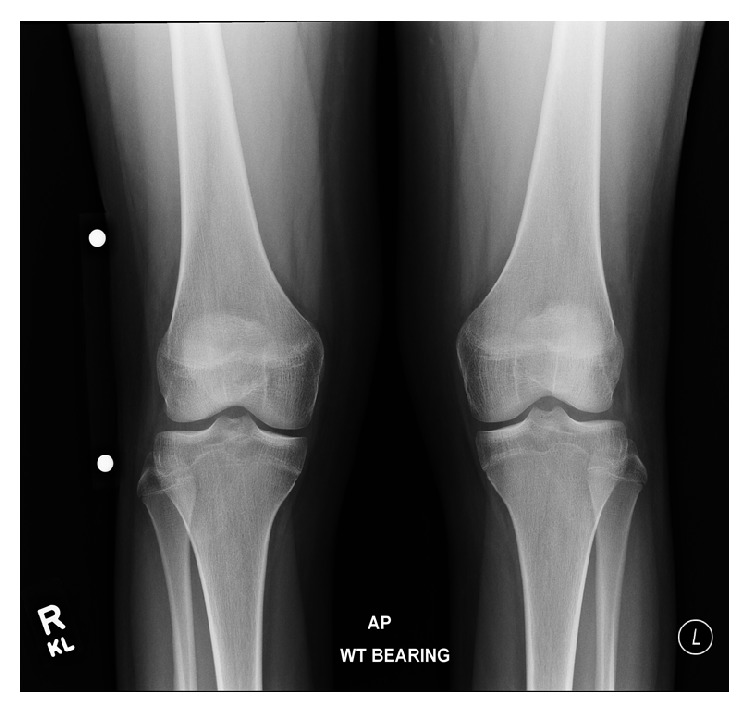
A 13-year-10-month-old female with FOPE zone of the distal right femur. Findings: presenting radiographs of the knees were normal. There is partial closure centrally of the bilateral proximal tibia physes. The bilateral distal femur physes are narrowed but remain open. Technique: AP radiograph kVp = 70, mAs = 8.
